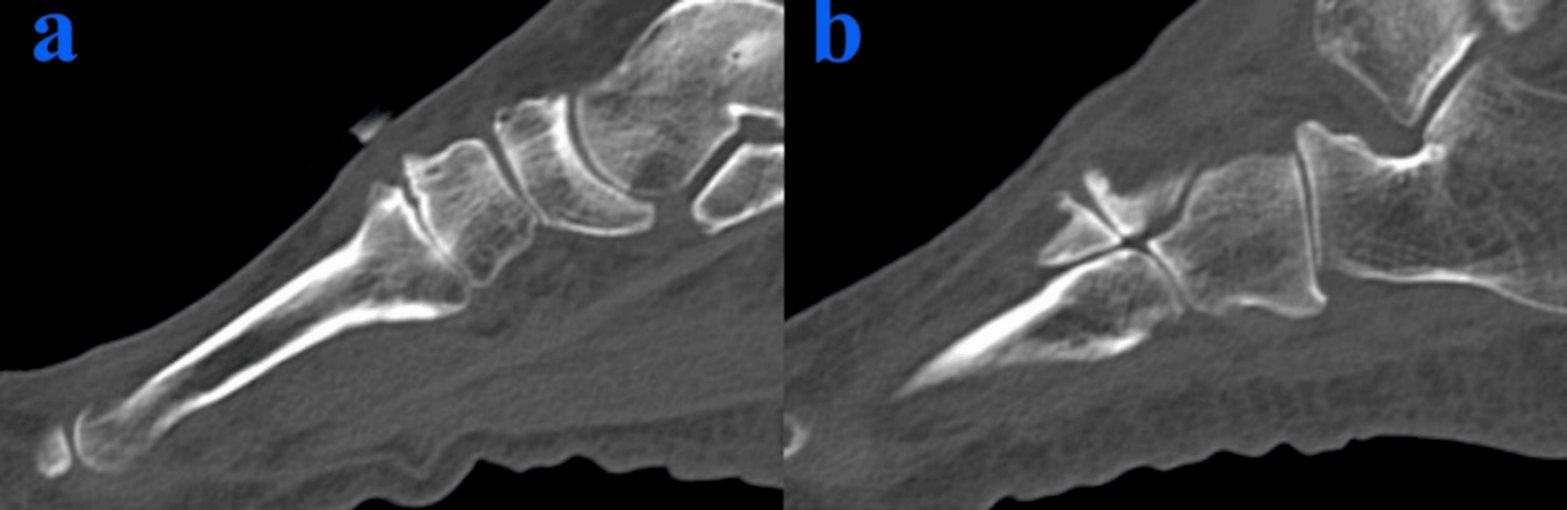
Preoperative CT scan (a) Second TMT joint; (b) third TMT joint TMT: tarsometatarsal The sagittal view of the images demonstrates osteophyte formation, commonly referred to as a dorsal boss. No significant articular changes and bone cysts were observed.
